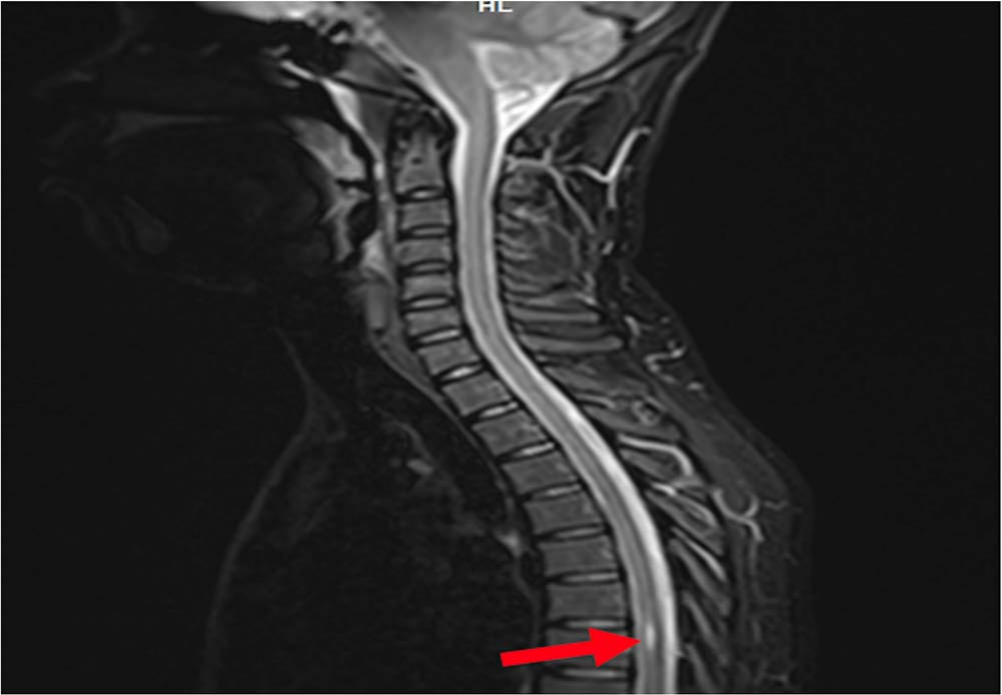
Sagittal T2-weighted MRI of the spinal cord performed for persistent pollakiuria in a 20-year-old man. A T2 hyperintense intramedullary lesion at the T6–T7 level is consistent with a syringomyelic cavity (red arrow) with no associated Chiari malformation.
